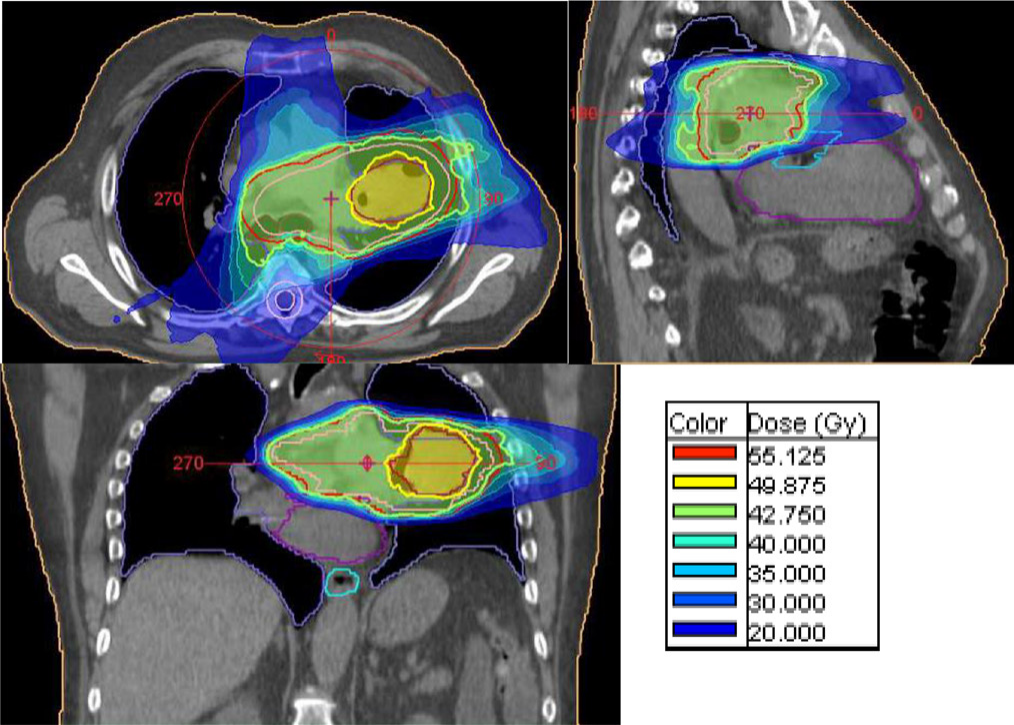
Images depicting the radiation plan used for treatment. Atelectasis occurred between planning-CT and start of irradiation.
